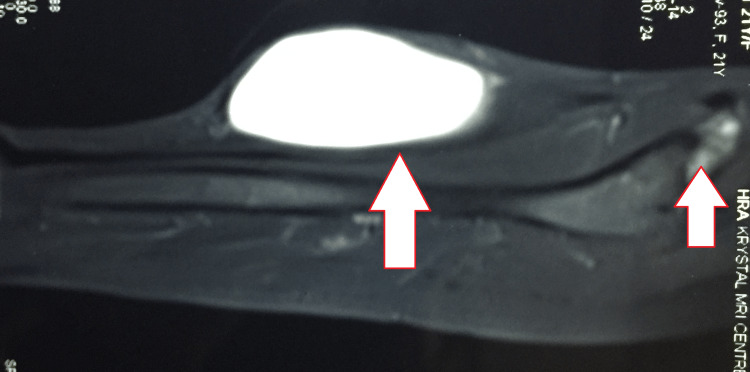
Magnetic resonance imaging of the pelvis and thigh
